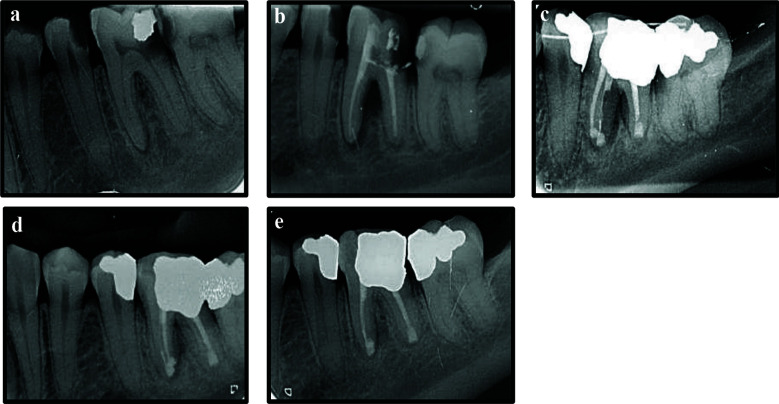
a, Preoperative periapical radiograph showing teeth 34 to 37; b, Non-surgical endodontic treatment in tooth 36 with separated instrument in mesiolingual canal extruding beyond the apex; c, Immediate post intentional replantation and splinting of tooth 36 to adjacent teeth; d, Six-months follow-up; e, Two years follow-up.
